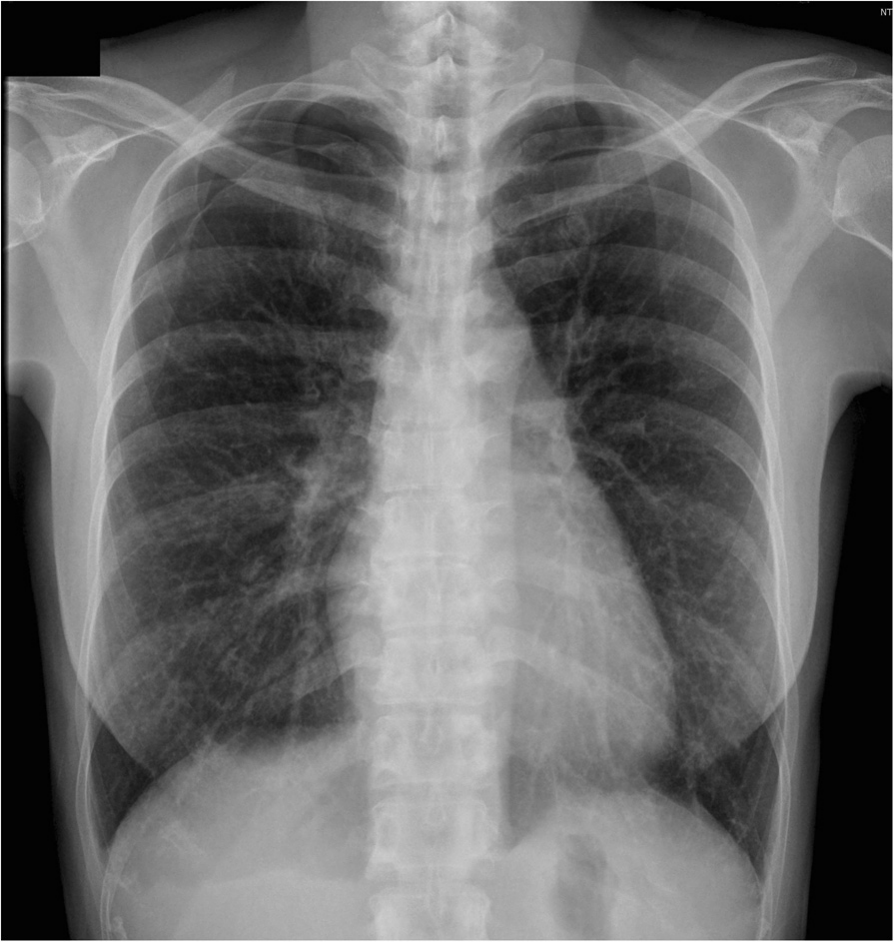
Chest plain film showed right pneumothorax with cystic change.
